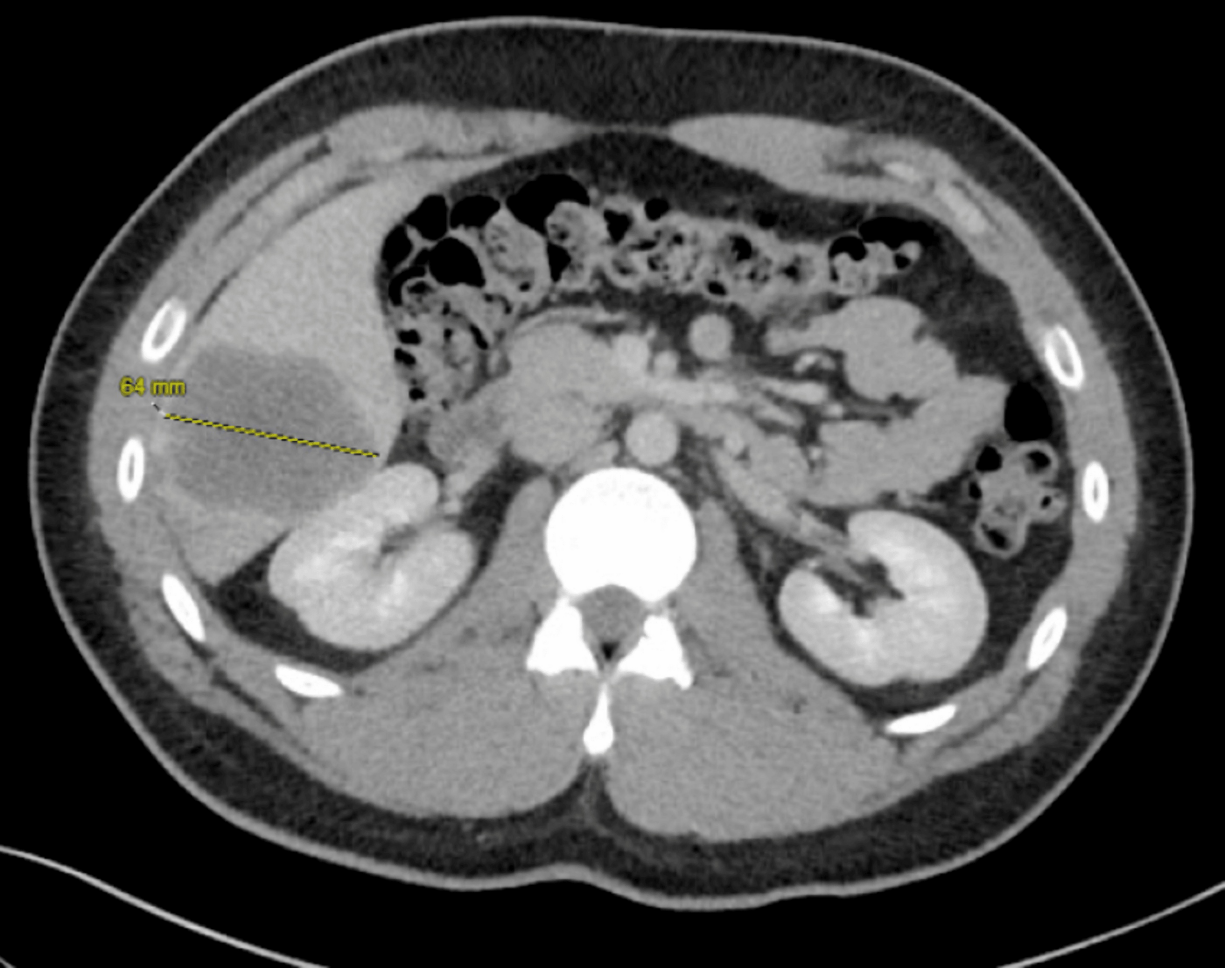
CT abdomen and pelvis (AP) demonstrating the largest liver metastasis, measuring 6.4 cm.
